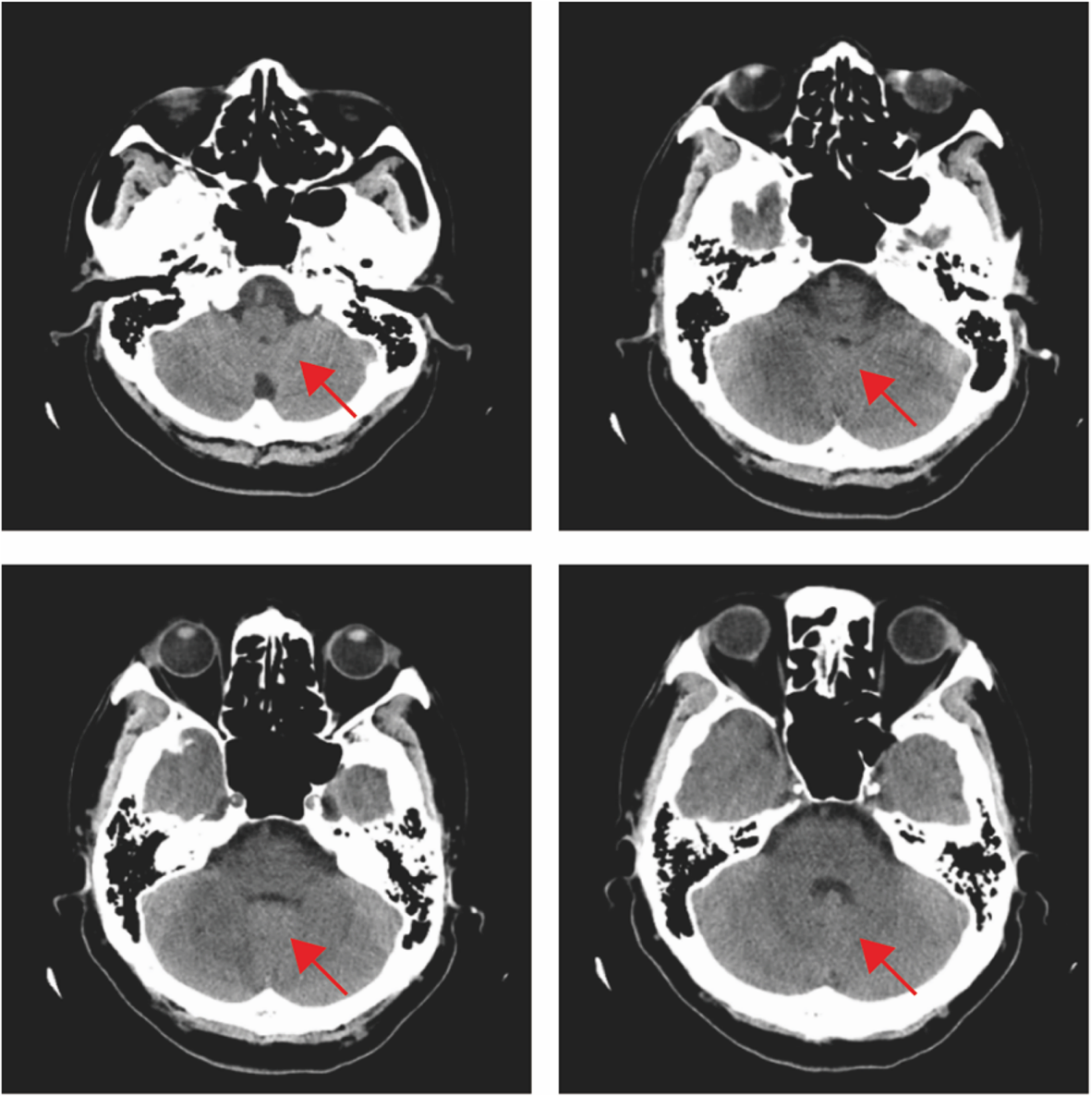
Plain head CT: bilateral cerebellar lesions were improved than before. Arrowheads: infection lesions
